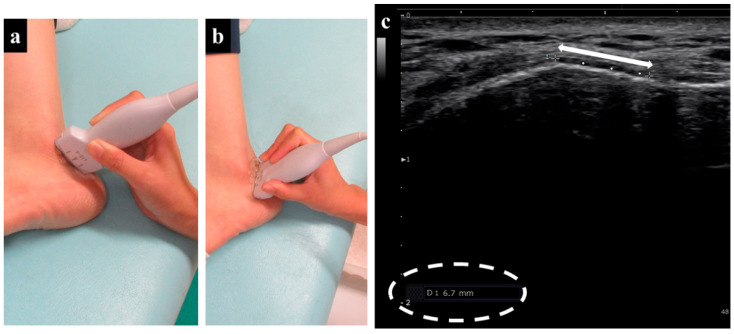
Method used to measure the width of the CFL using ultrasound imaging equipment: (a) after palpation, the CFL is extracted from the fibula to the talus in the long axial direction; (b) CFL width was measured by placing it in the short-axis direction at the midpoint; and (c) width measured in the short-axis image (6.7 mm). CFL: calcaneofibular ligament.
